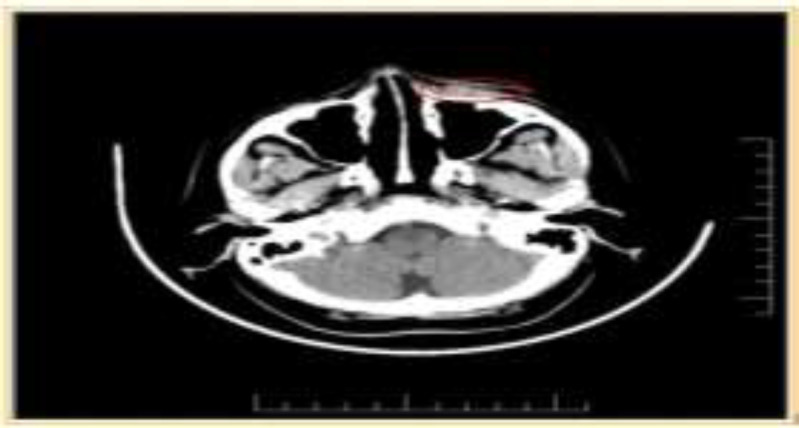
Showing CT axial cuts of lesion site, marked with red circle is the lesion present on left infraorbital region
